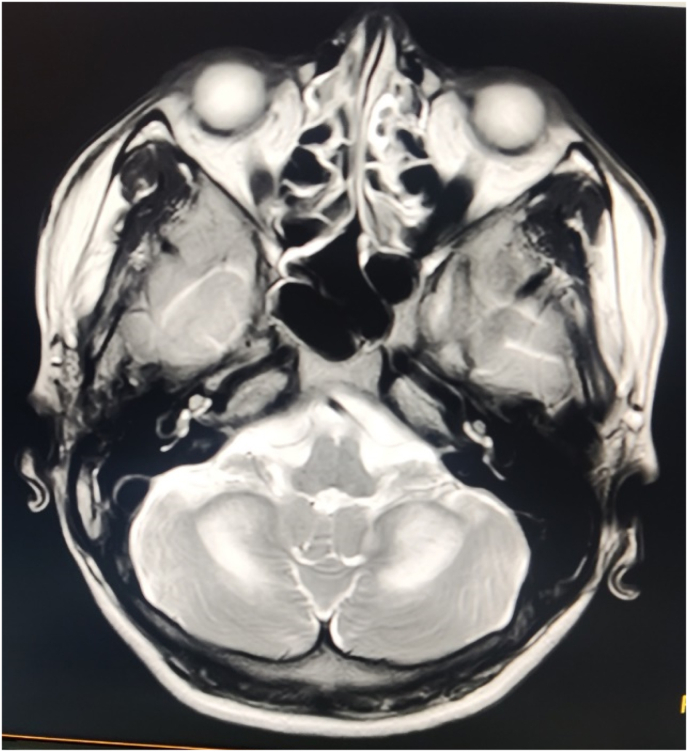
MRI scan of cerebellum showing hyper intensity along the medial aspect of the bilateral cerebellar hemisphere.
